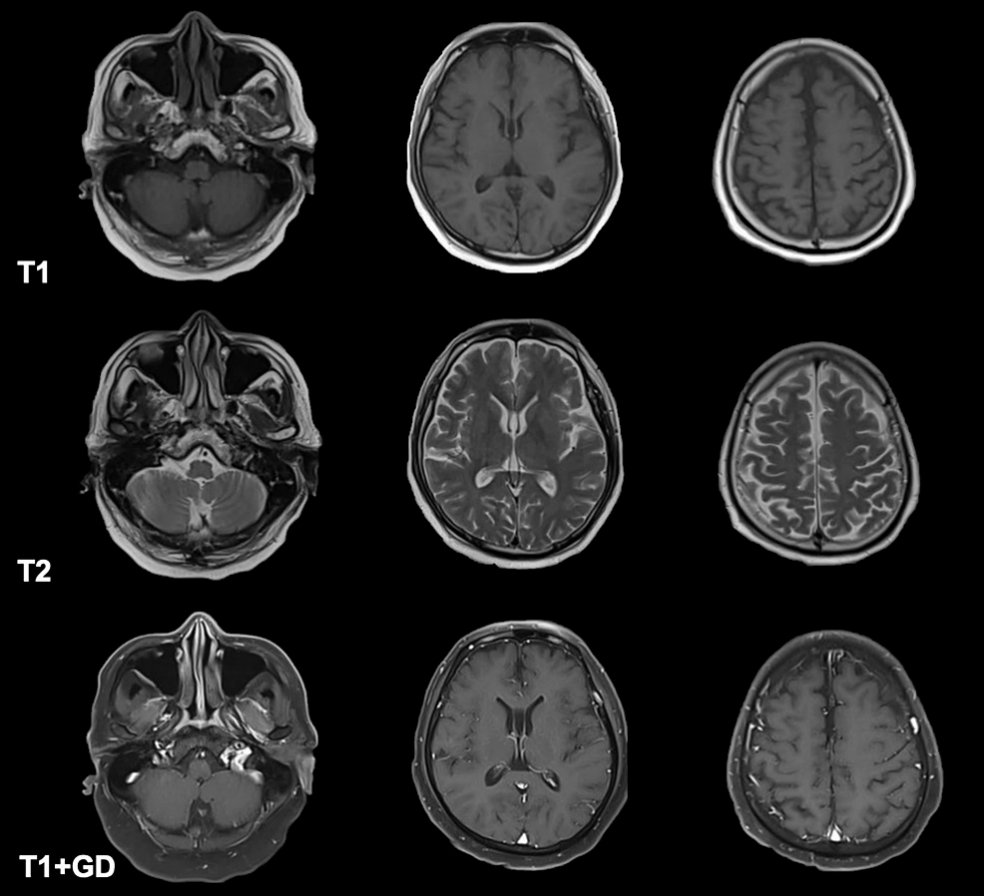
Cranial magnetic resonance imaging with contrast results Top row: axial T1 representative images, middle row: axial T2 representative images, bottom row: axial T1 with contrast representative images
